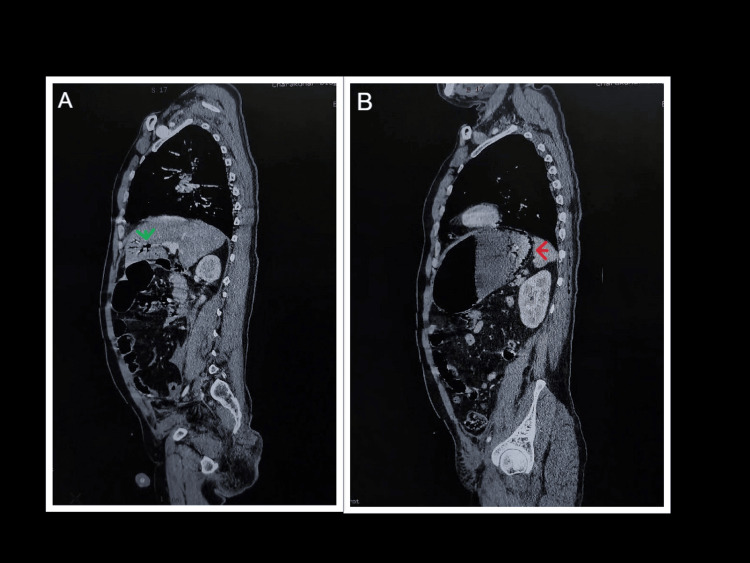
Sagittal section of CECT whole abdomen A. Portal venous gas marked with a green arrow. B. Gastric pneumatosis marked with a red arrow. CECT, contrast-enhanced computed tomography.
